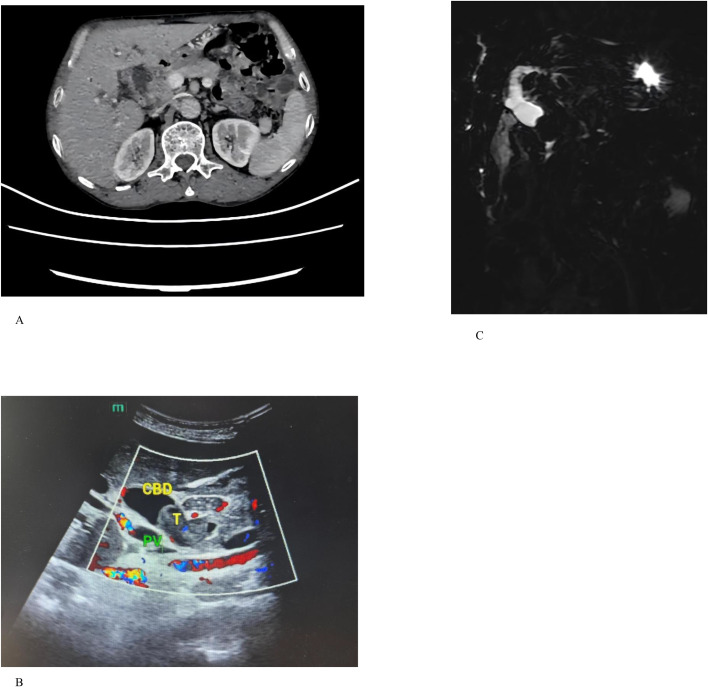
(A) CT examination reveals cholecystitis with dilation of the intrahepatic bile ducts, common hepatic duct, and proximal common bile duct; the middle and distal segments are poorly visualized. (B) Abdominal ultrasound demonstrates a space-occupying lesion in the common bile duct suggestive of pancreatic cancer, with associated intrahepatic and extrahepatic bile duct dilation. (C) MRCP demonstrates poor visualization of the gallbladder and cystic duct. Focal truncation of the pancreatic segment of the common bile duct (CBD) with an intraluminal filling defect is observed. The CBD, common hepatic duct, bilateral hepatic ducts, and intrahepatic bile ducts are dilated, without additional filling defects. The main pancreatic duct was not dilated.
